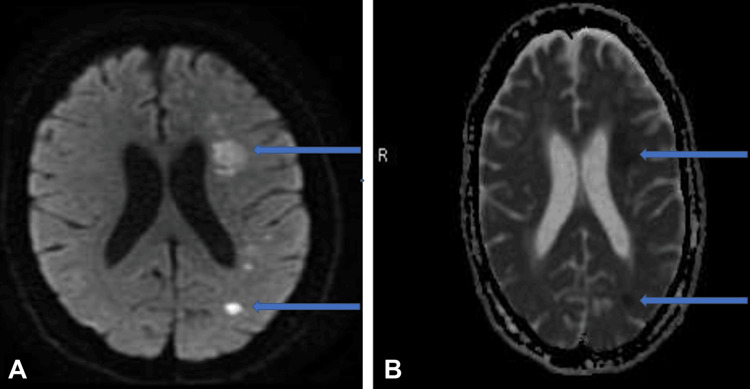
MRI brain (A) DWI and (B) ADC sequences showing multiple left hemispheric watershed infarcts (arrows) DWI: diffusion-weighted imaging; ADC: apparent diffusion coefficient
